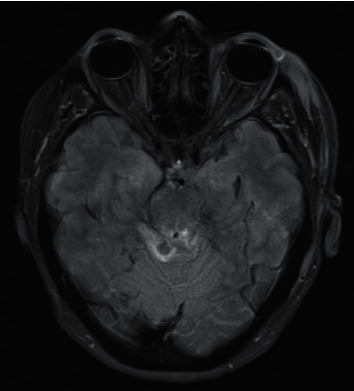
Axial FLAIR MRI showing Grade III DAI with hyperintense lesions at the level of the tectum of the midbrain, in the superior cerebellar parenchyma and the superior cerebellar peduncle.
